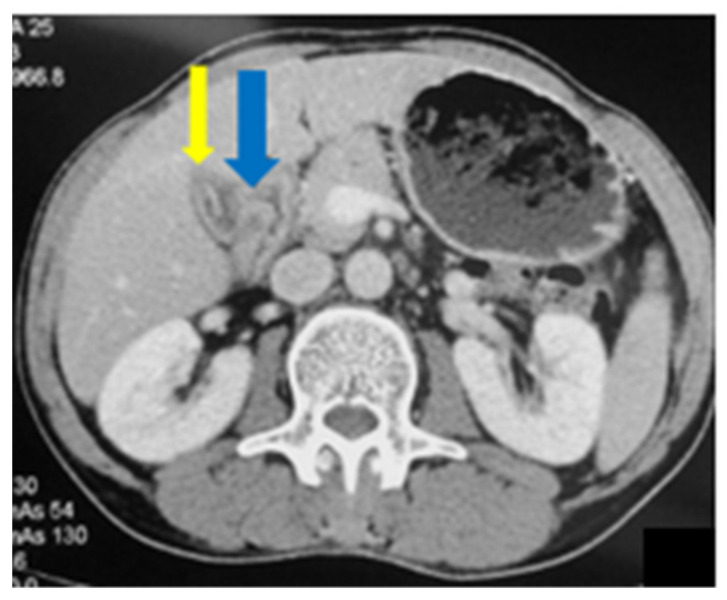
Abdominal CT: mild wall thickening of the gallbladder (blue arrow) with pericholecystic inflammatory changes (yellow arrow).
